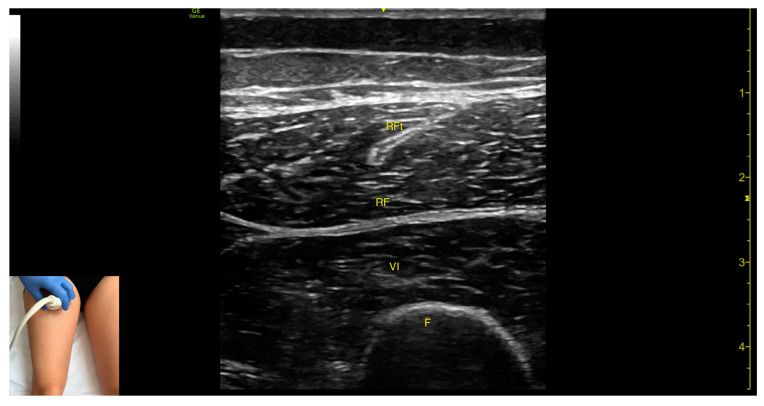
Ultrasound anatomy of *rectus femoris* (RF) with key landmarks: RF—*rectus femoris*; RFt—*rectus femoris* tendon; VI—vastus intermedius; F—femur.
